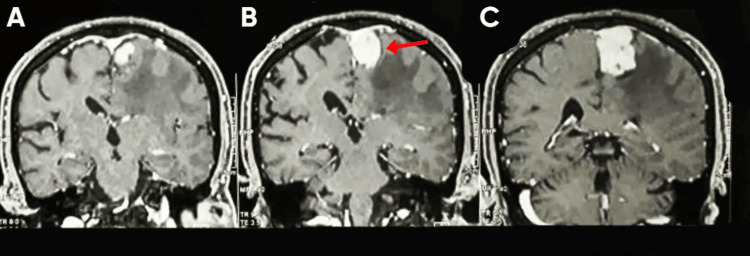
Preoperative coronal post-contrast T1-weighted MRI of the brain (A–C) Sequential coronal sections demonstrating a left parasagittal dural-based enhancing lesion. (B) The red arrow indicates the homogeneously enhancing meningioma compressing the adjacent motor cortex, consistent with a parasagittal meningioma.
